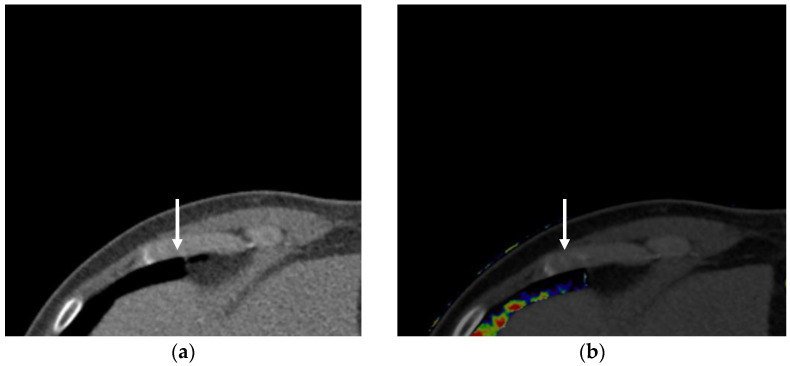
An acute pulmonary embolism: (a) CT pulmonary angiography; (b) Image of perfused blood volume in the lung. The clot (arrow) in the pulmonary artery is difficult to identify because of the lack of spatial resolution in CT pulmonary angiography. The image of the perfused blood volume images shows the perfusion defect in the affected lung area (arrows), which can improve diagnostic accuracy for detecting a pulmonary embolism.
